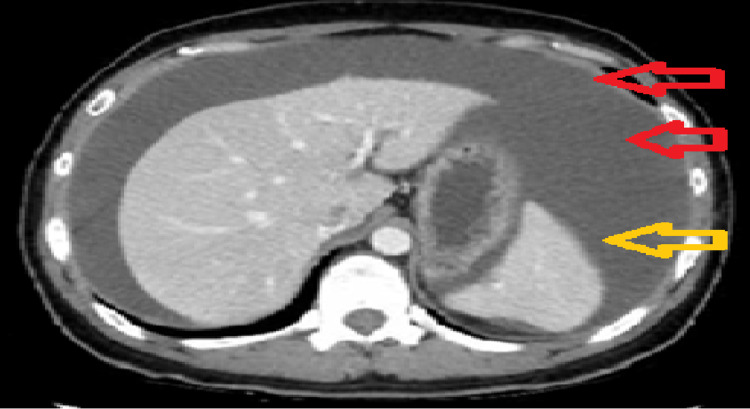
CT abdomen reveals huge ascitic fluid concentration (red arrows) that led to the push-down of the spleen to the level of the backbone (yellow arrow) CT: computed tomography
